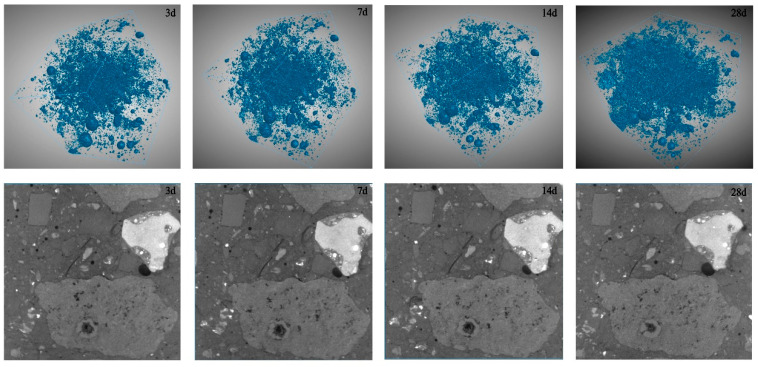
X-CT Image of SCC with 3% CAI dosage.
